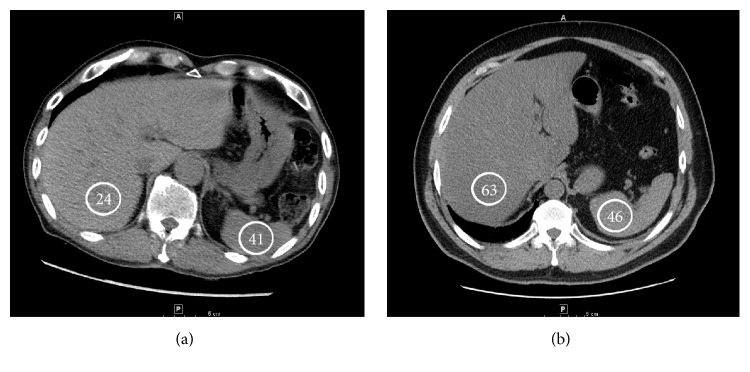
CT images showing an example of steatosis (a) and a nonsteatotic liver (b). Hounsfield Units are indicated in the circles.
